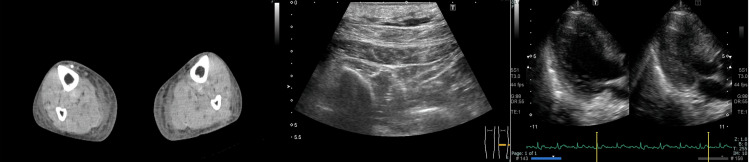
Imaging studies during hospitalization. Non-contrast computed tomography of the lower extremities (left) demonstrated diffuse soft tissue edema without gas formation or abscess, making necrotizing soft tissue infection unlikely.
